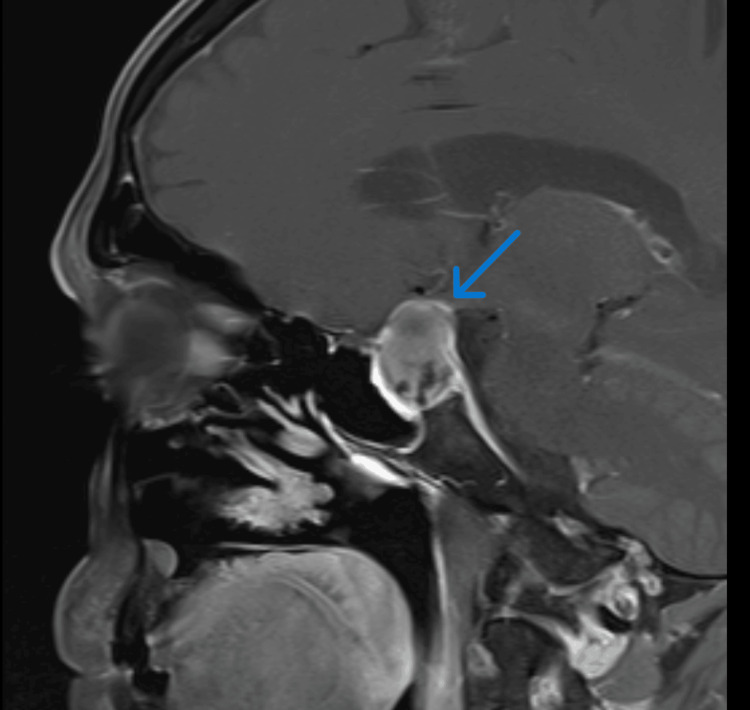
MRI showing enlarged pituitary gland with heterogenous internal signal intensities in keeping with pituitary apoplexy
